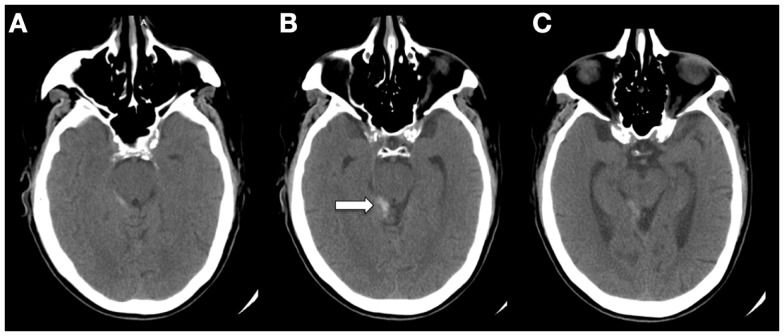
CT (A–C) demonstrating SAH in the quadrigeminal and supracerebellar cisterns (arrow). The image was taken 48–64 h after minor trauma to the head while the patient was anticoagulated with warfarin. Although this is not a classic traumatic location for SAH, redistribution may have occurred between the time of trauma and when the image was taken.
